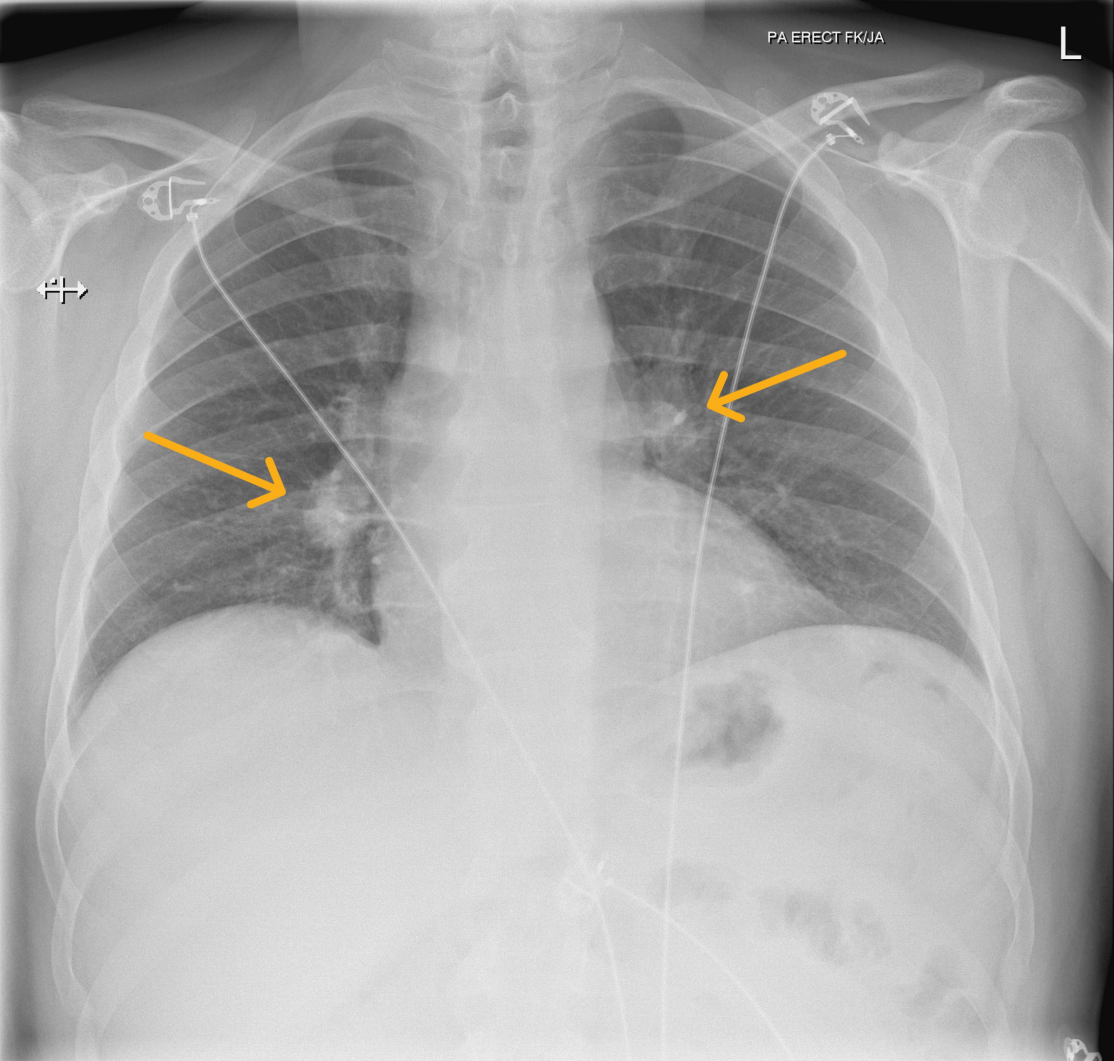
Chest X-ray with arrows showing symmetrical hilar and mediastinal lymphadenopathy.
